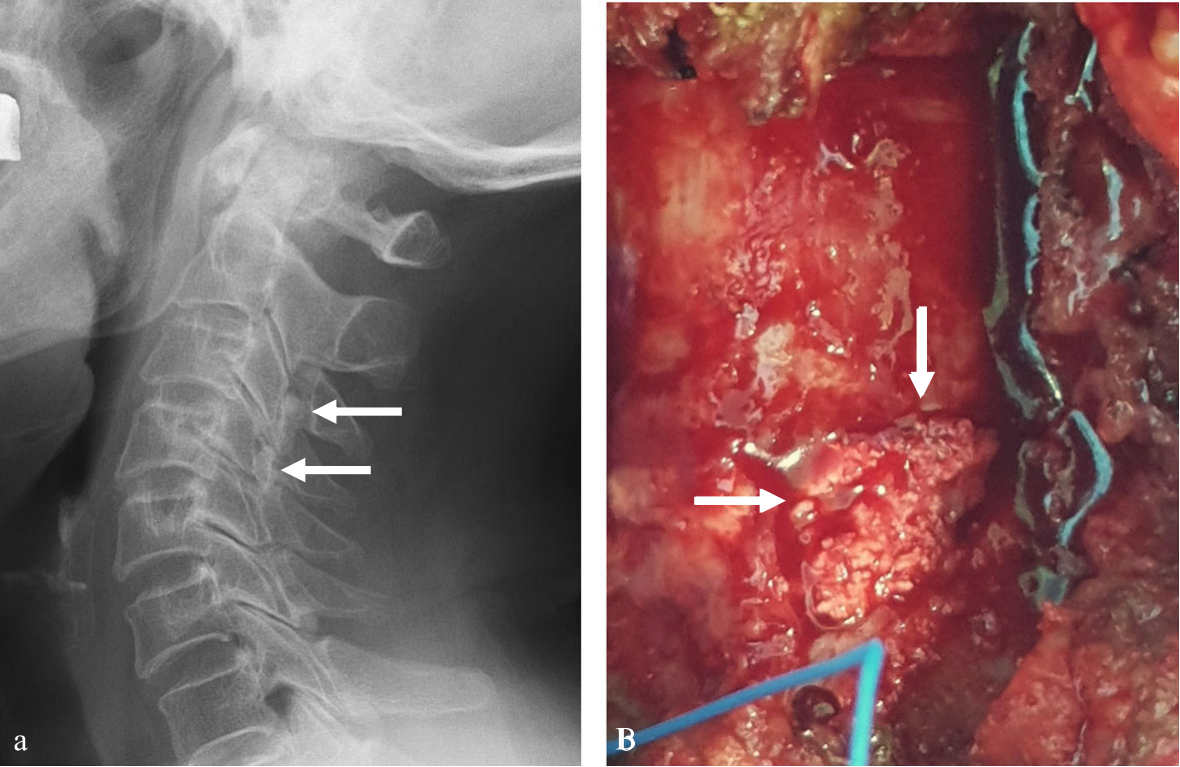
Lateral radiograph (a) of the cervical spine showed round calcified lesions at the laminae of C3 and C4 (white arrows). Intraoperative clinical photo (b) revealed CPPD deposition after total laminectomy of C3 and C4 (white arrows)
